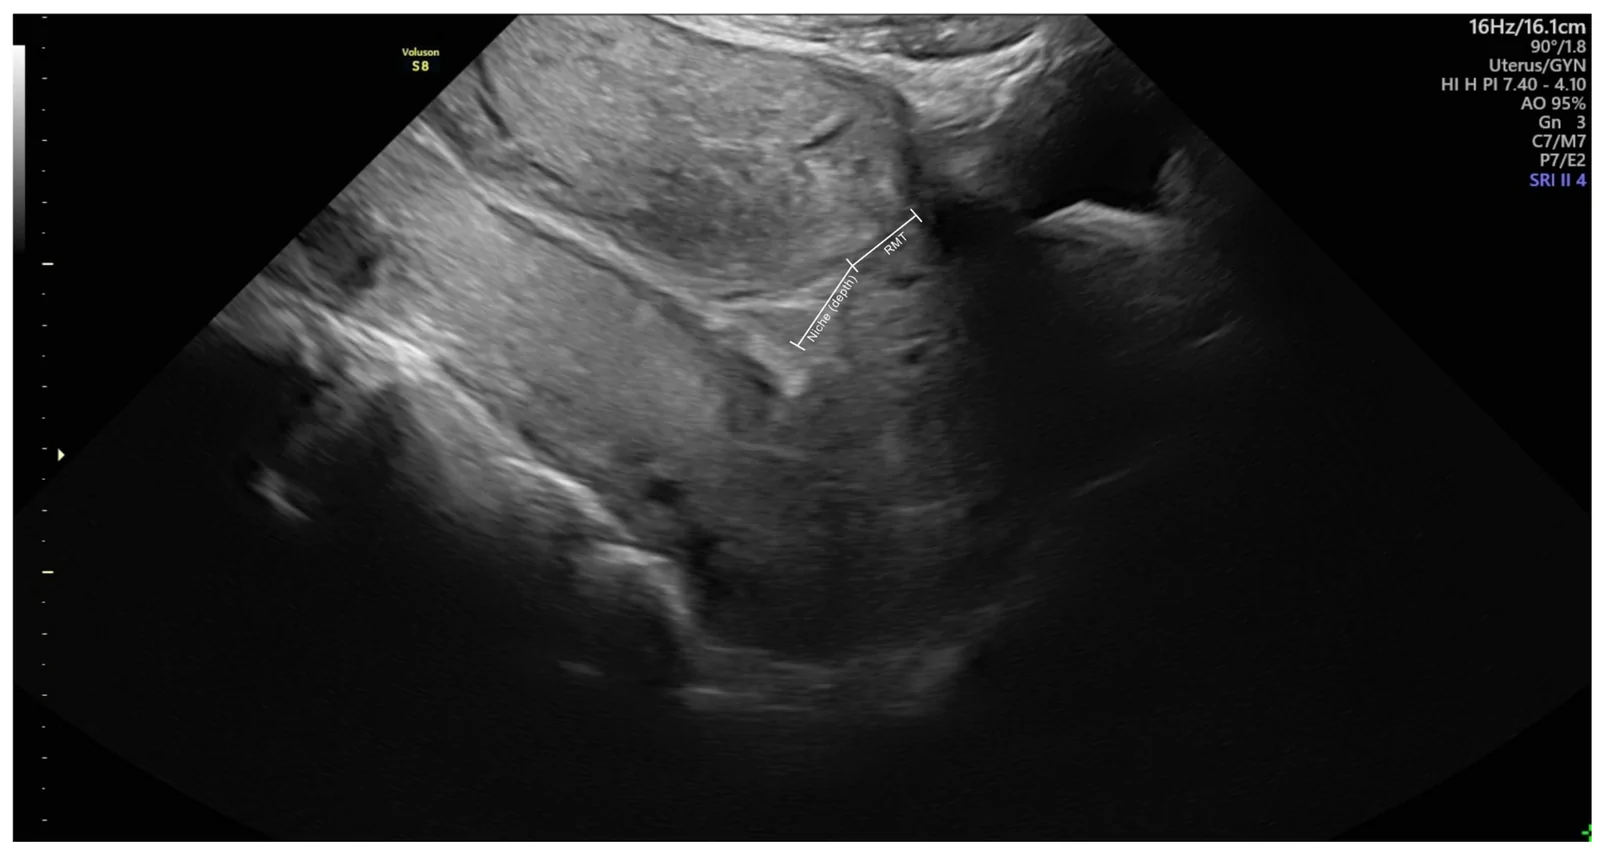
Uterus of a patient in sagittal section using transabdominal sonography (TAS). The lower uterine segment with a uterine defect after vaginal birth after caesarean section (VBAC) can be seen.
